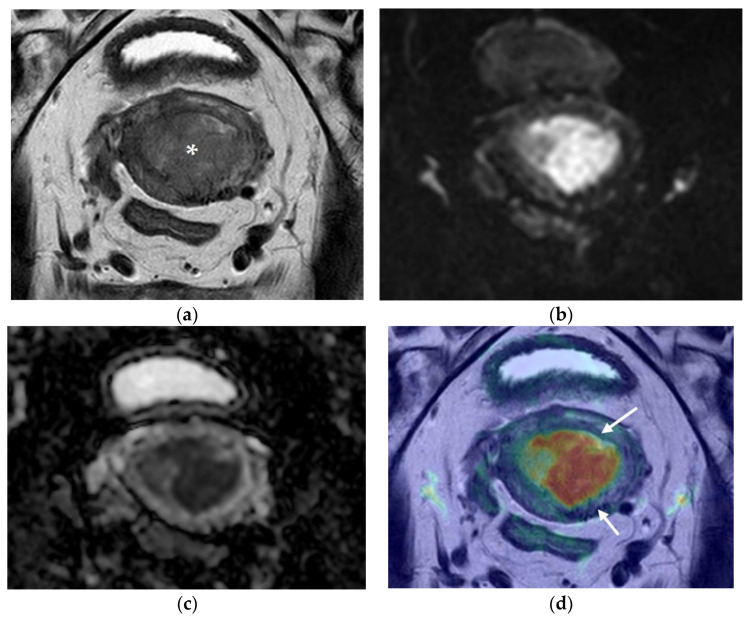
Pelvic MRI. Axial oblique sequences oriented to the corpus uteri long axis: (a) T2WI; (b) DWI (b1000); (c) ADC map; (d) fused T2WI-DWI image. Endometrial cancer is perceptible in (a) as a polypoid lesion with posterior implantation base (asterisk). When interpreting the morphologic (a) and functional images (b,c), deep myometrial invasion is questionable, but clearly excluded in fused images (d), where clear demarcation of the endometrial cancer and external myometrium is evident (arrows).
